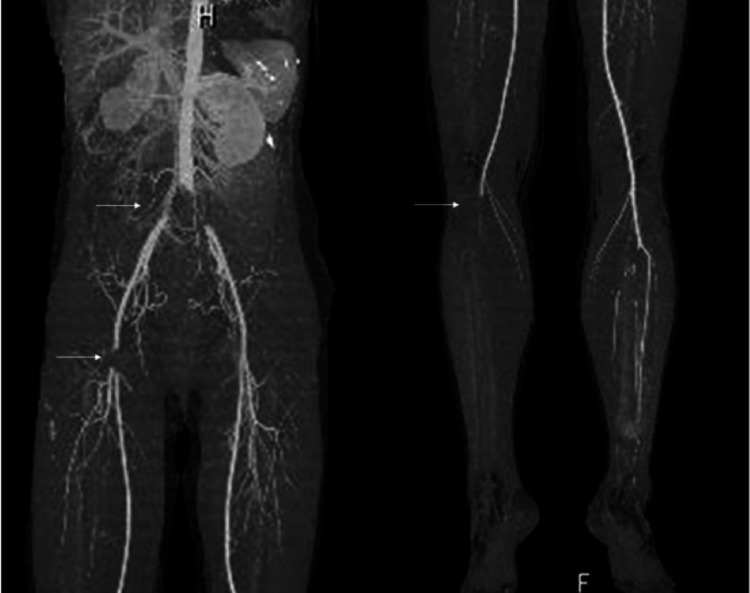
Pre-operative contrast-enhanced CT CT reveals the occlusion of the abdominal aorta, left and right common iliac arteries, right common femoral artery, and right patellar artery, as indicated by arrows. CT, computed tomography
